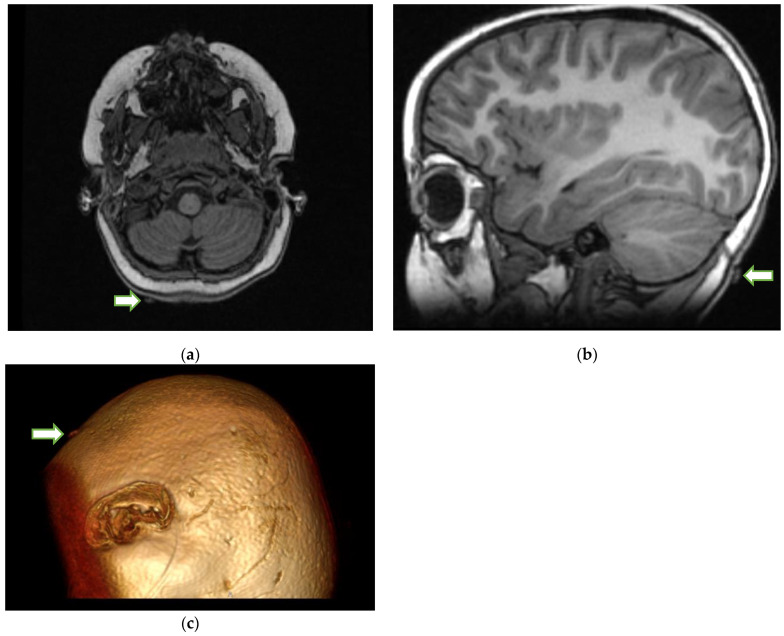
Axial T1-weighted (a) MRI of the brain at the level of the medulla shows a normal appearance of the medulla and the cerebellum. However, on closer inspection, there is a small, isointense area (arrow) seen attached to the skin in the occipital region on the right side. The arrow on the sagittal T1-weighted MRI of the brain (b) also points to the tick. The 3D rendering of the MRI (c) shows what appears to be a tick (arrow) on the surface of the head.
